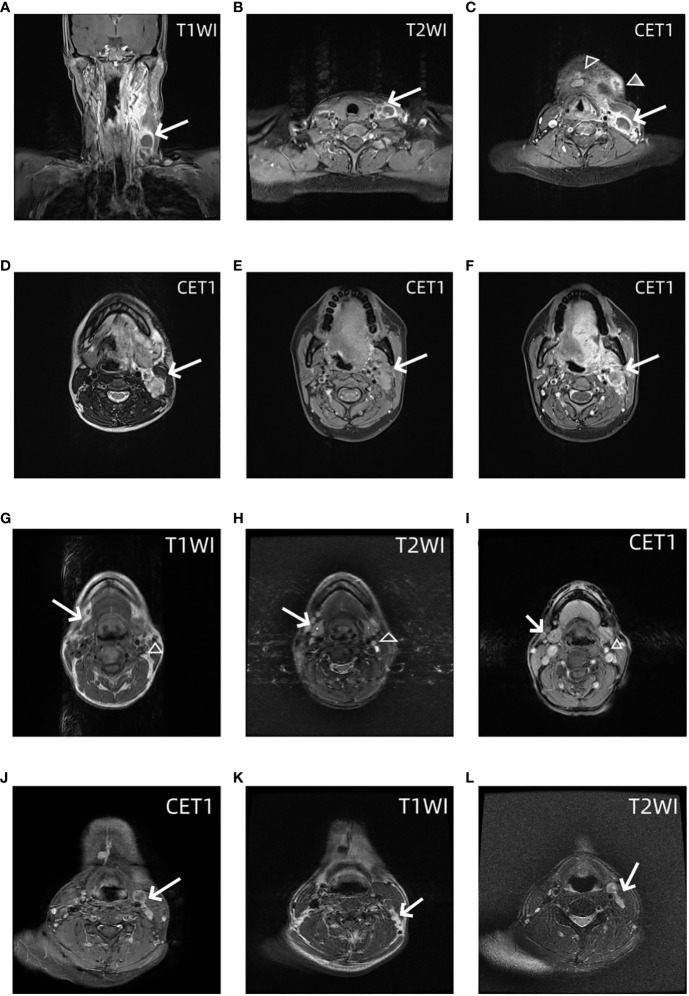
MRI images of the lower neck of a patient with a T4 stage OTSCC. (A) Coronal contrast enhanced T1 weighted images shows Levels III and IV lymph nodes have macroscopic metastasis (arrow). (B) Axial contrast enhanced T1 weighted images show Levels IV lymph nodes have macroscopic metastasis (arrow). (C) Axial contrast enhanced T1 weighted images show Levels I (trangle) and III lymph nodes (arrow) have macroscopic metastasis. MRI images of the upper neck of the same patient. Axial T1 weighted images (D) and T2 weighted images (E) and contrast enhanced T1 weighted images (F) show Levels II lymph nodes have macroscopic metastasis (arrow). MRI images of the neck of OTSCC patients with stage T3 occult lymph node metastasis. Axial T1-weighted images (G), T2-weighted images (H), and T1-enhanced images (I) show grade I and grade II occult lymph node metastases (empty triangle and arrow), that is, normal lymph nodes were diagnosed on MRI, but lymph node metastases were found on pathology. In another patient, axial T1 enhanced images (J), T1-weighted images (K), and T2-weighted images (L) show grade III and IV occult lymph node metastases (empty triangle and arrow).
